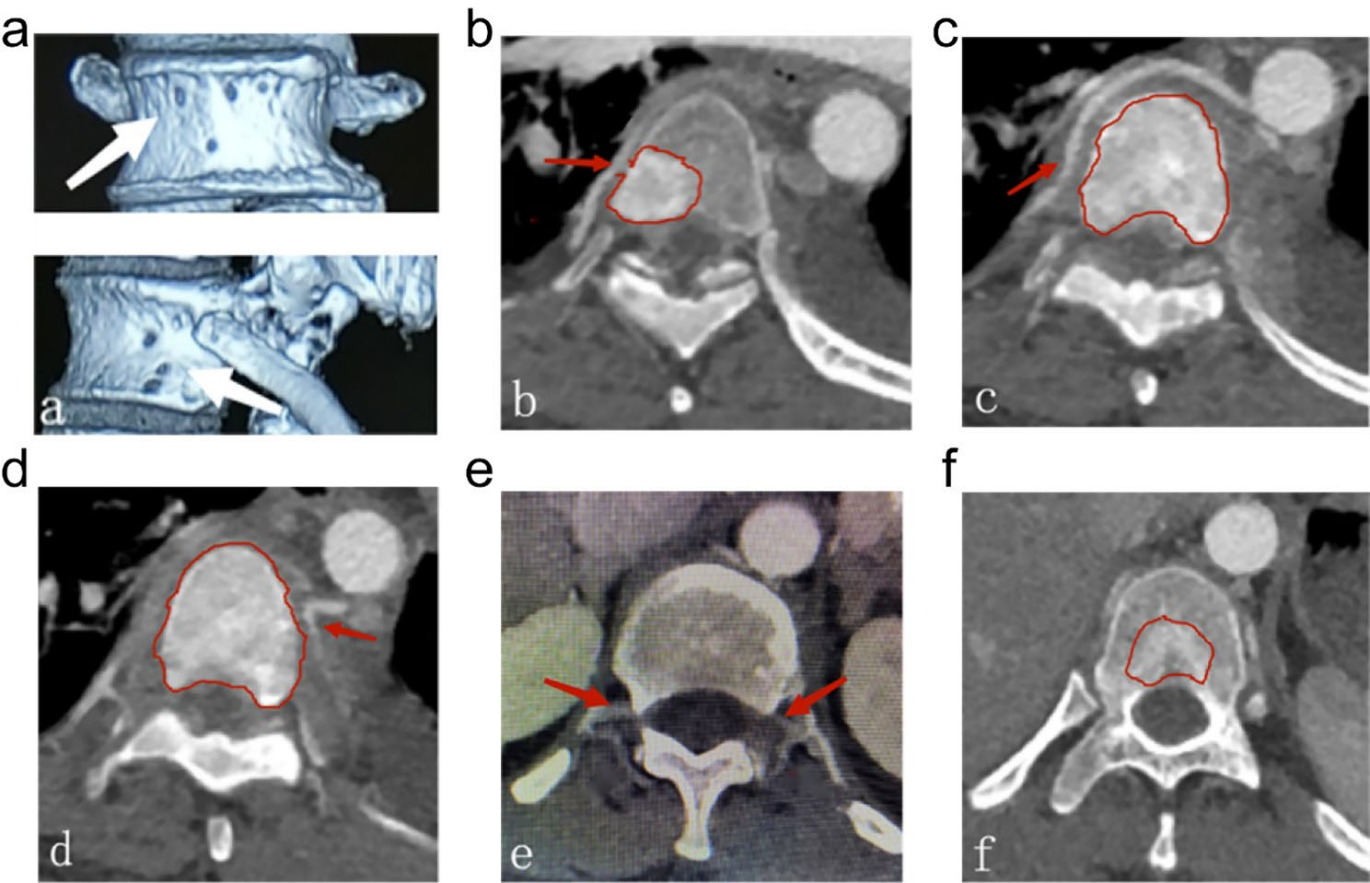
The vascular territory of the vertebral body. (a) Pores of vertebral arteries in nutrient arteries anterior to the vertebral body, periosteal arteries, and rete-like arteries in metaphysis (white arrow). (b) Enhanced CT showed the nutrient artery derived from the right segmental artery extended towards the vertebral body (red arrow) and areas of blood supply to the tumor in vertebral body (red outline). (c) Enhanced CT showed the nutrient artery originated from the right segmental artery was directed to the vertebral body (red arrow) and areas of blood supply to the tumor in vertebral body (red outline). (d) Enhanced CT showed the nutrient artery was sent from the left segmental artery to the vertebral body (red arrow) and areas of blood supply to the tumor in vertebral body (red outline). (e) Enhanced CT showed bilateral segmental arteries, posterior branches, spinal branches and anterior branches of vertebral canal formed the nutrient arteries that anteriorly supplied the blood flow of posterior vertebral body (red arrow). (f) Enhanced CT showed areas of blood supply to the tumor in the posterior vertebral body (red outline)
